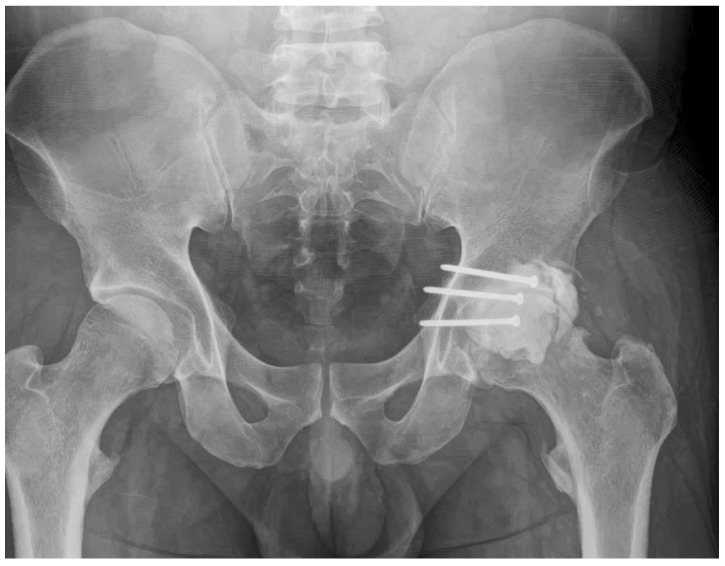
Twenty-one-month postoperative follow-up AP pelvic radiograph. The image shows no evidence of screw loosening and a well-maintained joint space.
